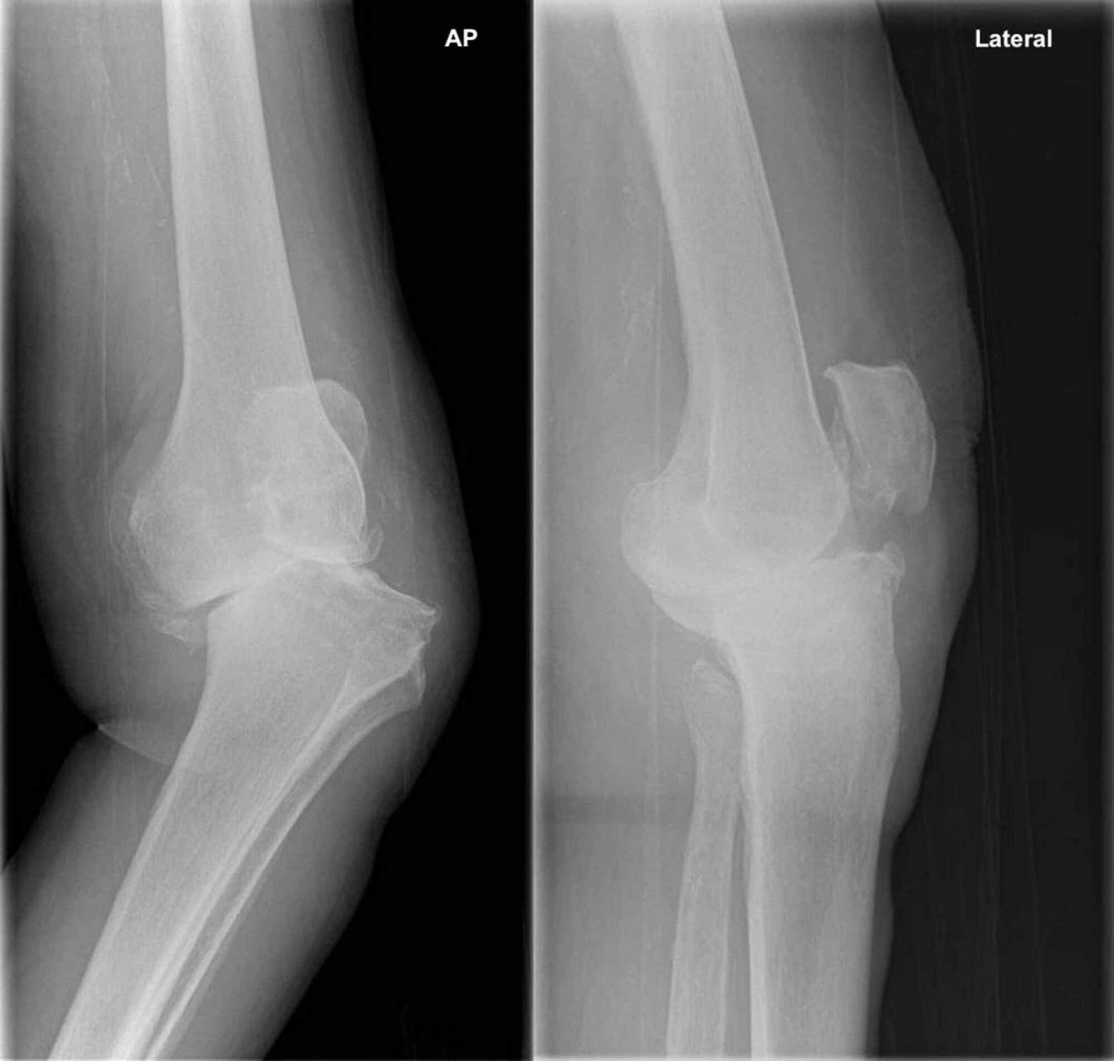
Anterior-posterior and lateral radiographs of the left knee revealing end-stage osteoarthritis with varus deformity.
